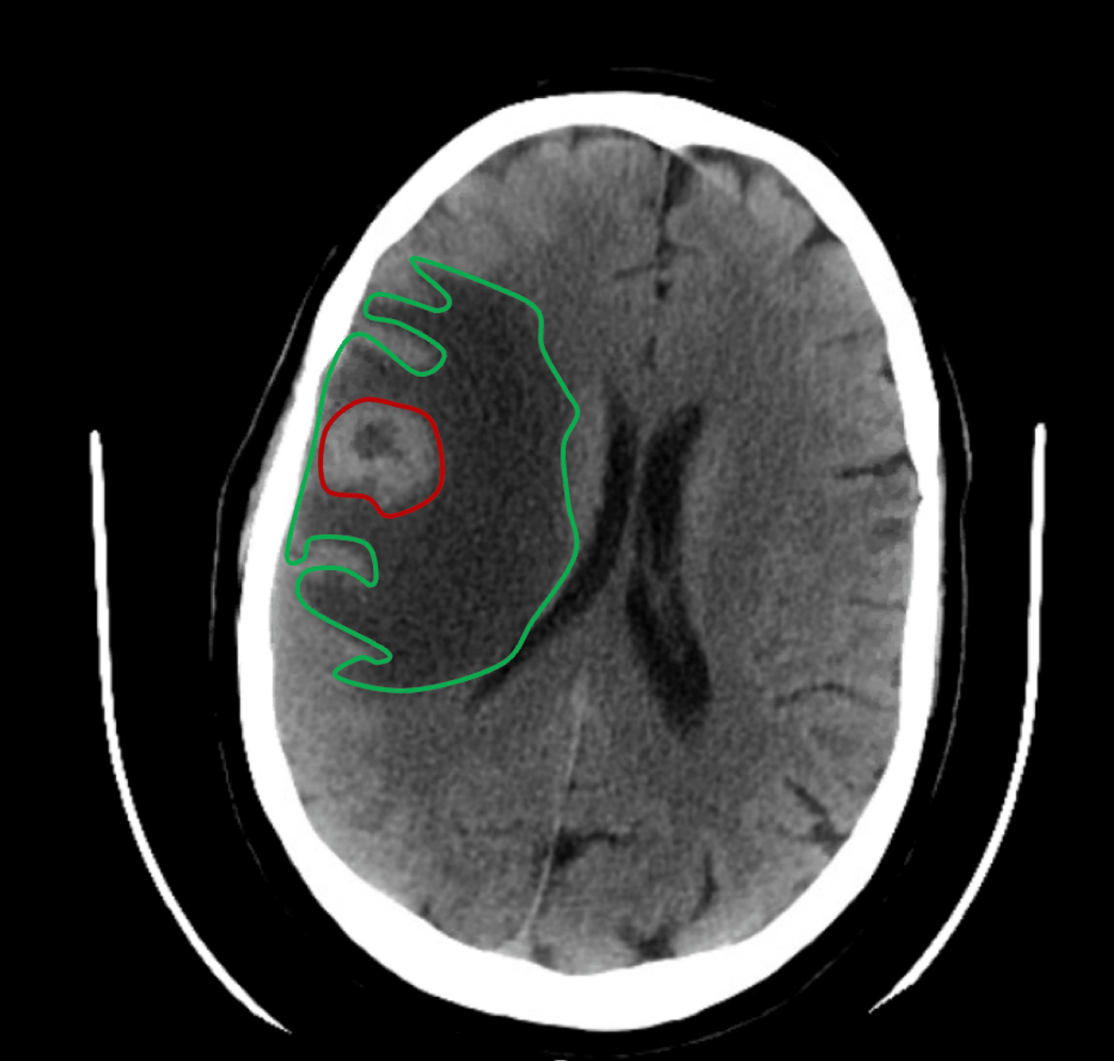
Non-contrast head CT showing a right frontal lesion (outlined in red). A non-contrast computed tomography (CT) scan shows a well-defined, round, space-occupying lesion in the left frontal corticosubcortical region (red circle), measuring 2.6 × 2.7 cm. Associated frontoparietal edema (green circle) extends to the ipsilateral internal and external capsules, causing a 6 mm leftward midline shift.
